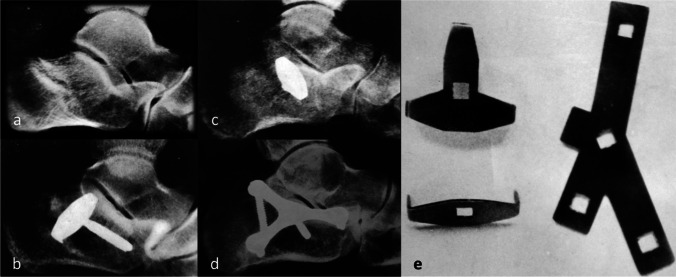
Radiographs of multi-fragmentary thalamic fractures treated with different synthesis systems: a) example of a thalamic fracture with sinking fragment; b–c) reduction and synthesis with a straight plate with two anchor points and a screw d) reduction and fixation with a Y-screw plate and screws; e) straight plates with two and three anchor points respectively (to the left), and a Y-screw plate (to the right) used at Orthopaedic Department of Padua, 1965
